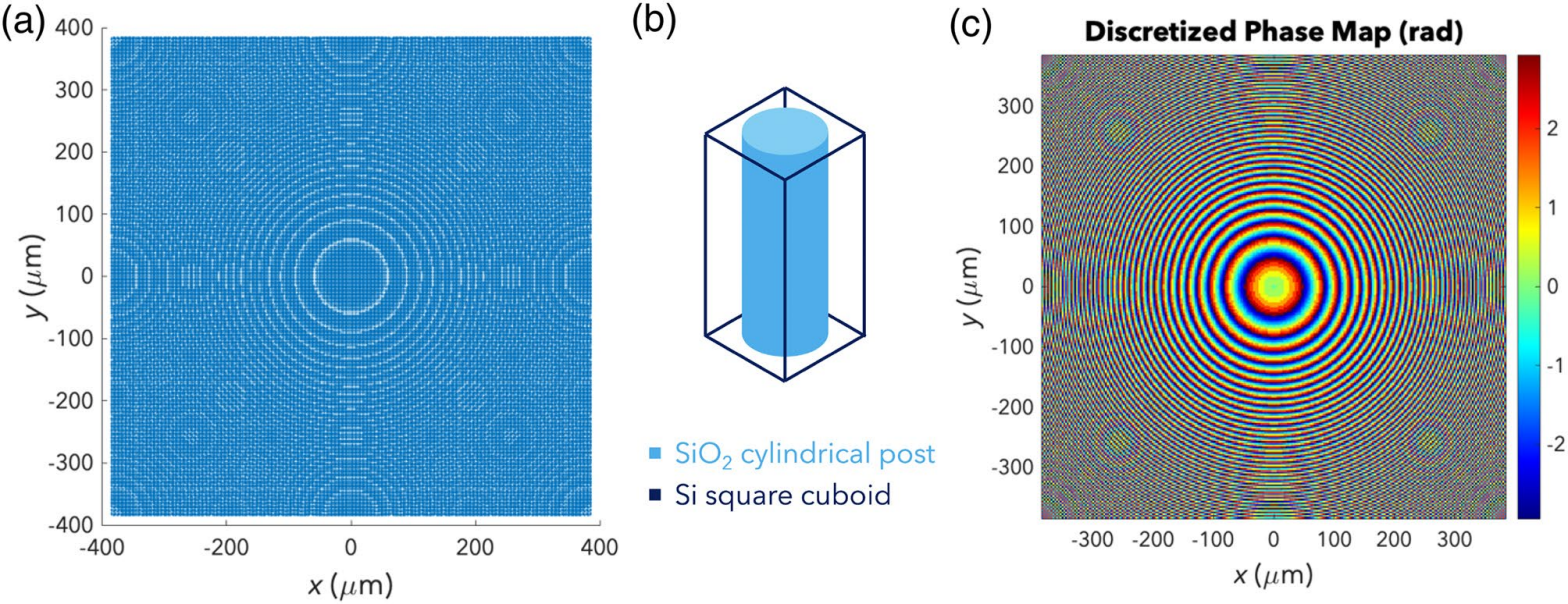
Ultrasonic metalens. (a) Top view of the ultrasonic metalens. (b) Unit cell made up of a SiO_2_ cylindrical post (light blue) embedded in a Si square cuboid (dark blue). (c) Discretized phase map of the metalens.
